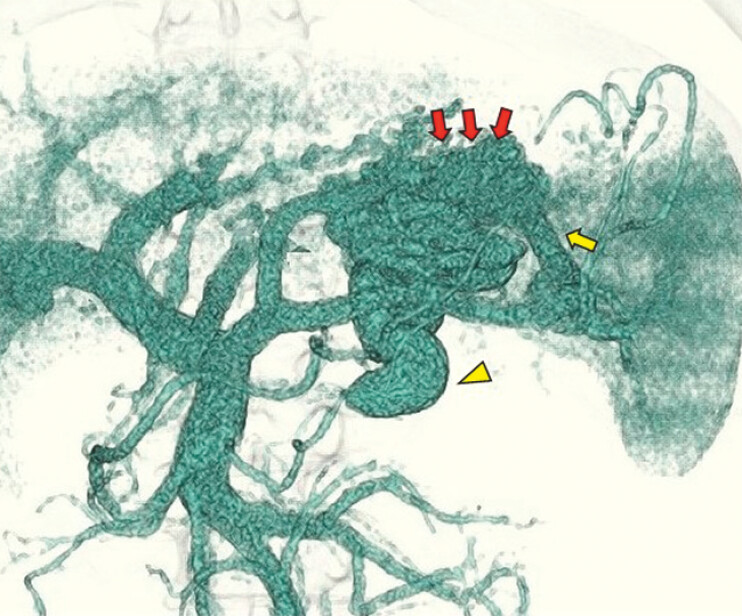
Three-dimensional contrast-enhanced computed tomography revealed the hemodynamics of the varices (red arrows), showing that they were fed from the short gastric vein (yellow arrow) to the renal vein (yellow arrowhead).
